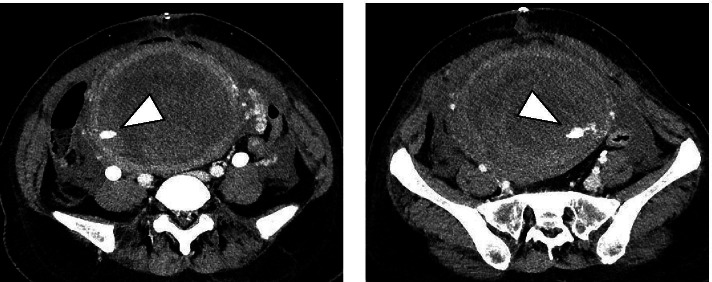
Contrast-enhanced computed tomography images. A computed tomography scan
